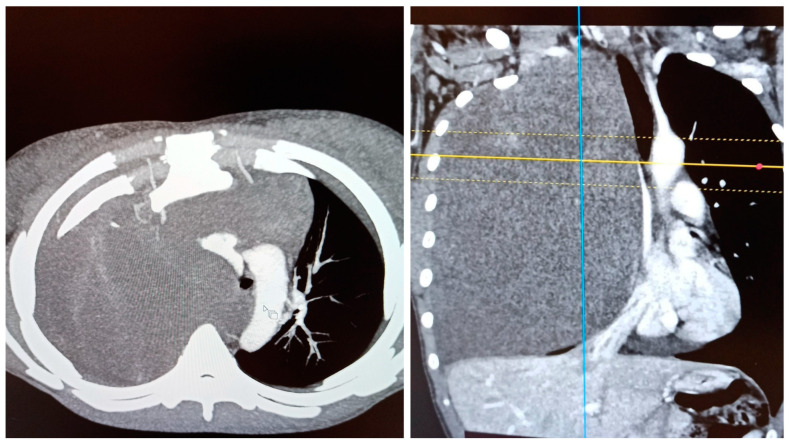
Preoperative CT-scan images of the tumor.
